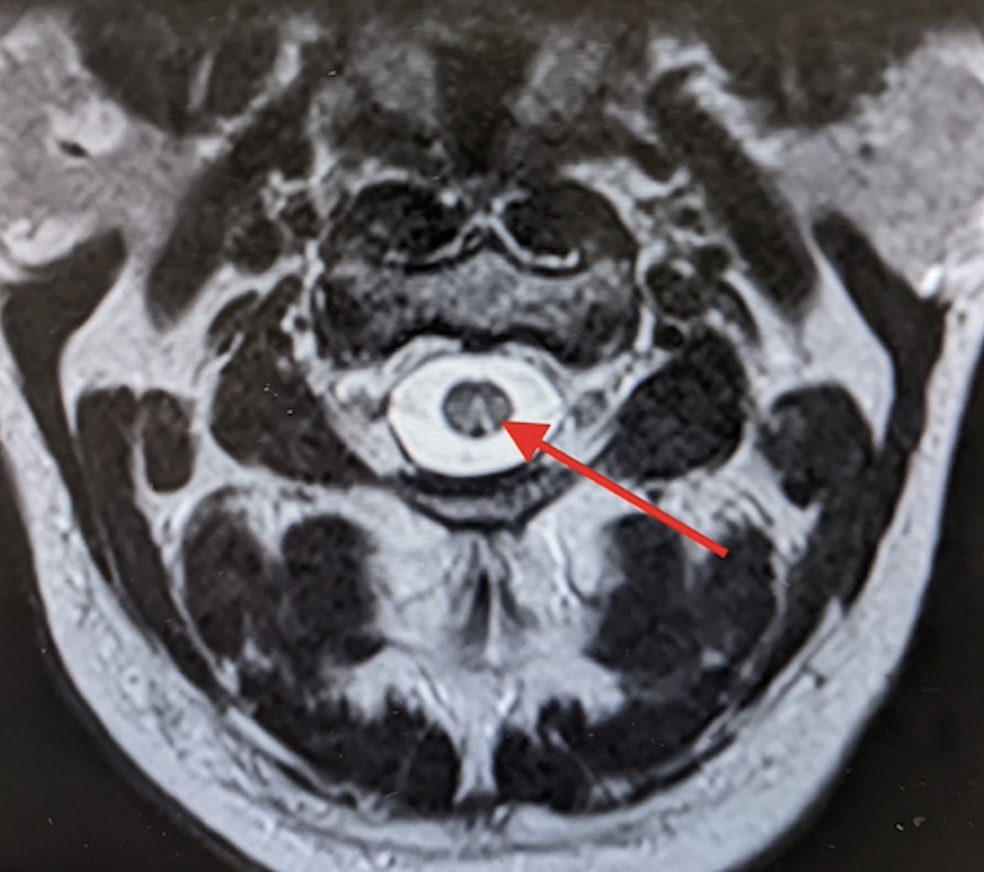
MRI axial cervical spine showing the “inverted V sign” (red arrow), consistent with SCD of the cord. MRI: Magnetic Resonance Imaging; SCD: Subacute Combined Degeneration.
